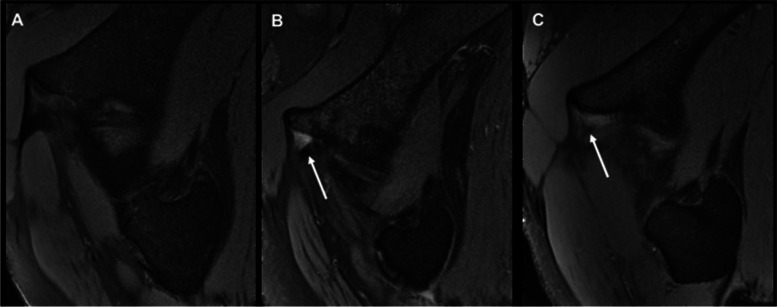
Normal Anterior Inferior Iliac Spine (A) and Superior Capsular Oedema in an AIIS on MRI (B-C)
